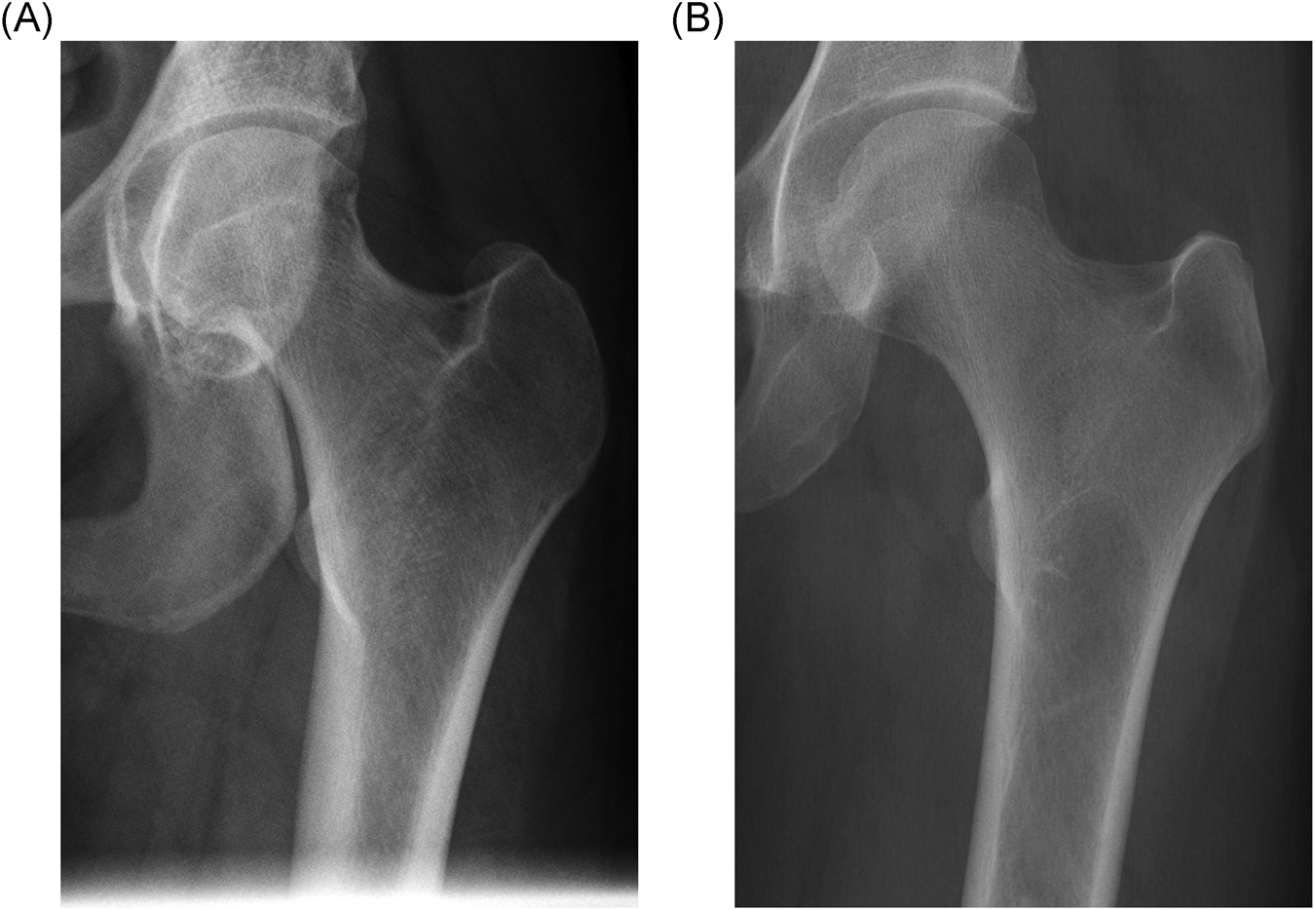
Plain radiographs of the proximal left femora of two patients with Gaucher disease. (*A*) Normal cortical thickness in the femoral shaft of the left proximal femur. (*B*) Reduced cortical thickness in the femoral shaft of the left proximal femur. Note the thinner cortex in comparison with *A*
